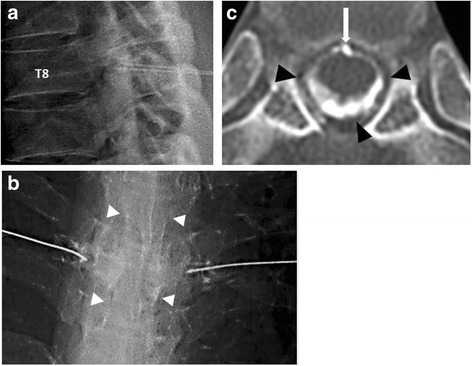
a Fluoroscopy-guided transforaminal EBP through bilateral T8 nerve roots (lateral view. b After contrast medium injection to confirm the epidural space (*white arrowheads*) (anteroposterior view. c Post-TFEBP spinal CT image at T7/T8 disc level demonstrating extension of injected blood through the entire epidural space (*black arrowheads*).The *white arrow* shows a partially calcified disc
